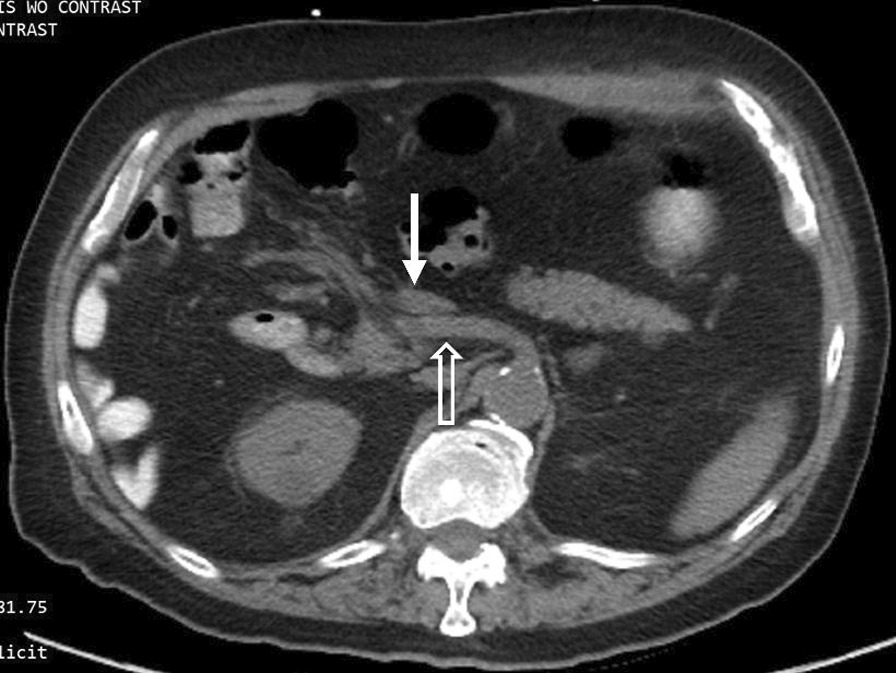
SMA-SMV relationship. CT of the abdomen without intravenous contrast and with positive oral contrast. The superior mesenteric artery (hollow arrow) is abnormally positioned to the right of the superior mesenteric vein (solid arrow) near the mesenteric root
